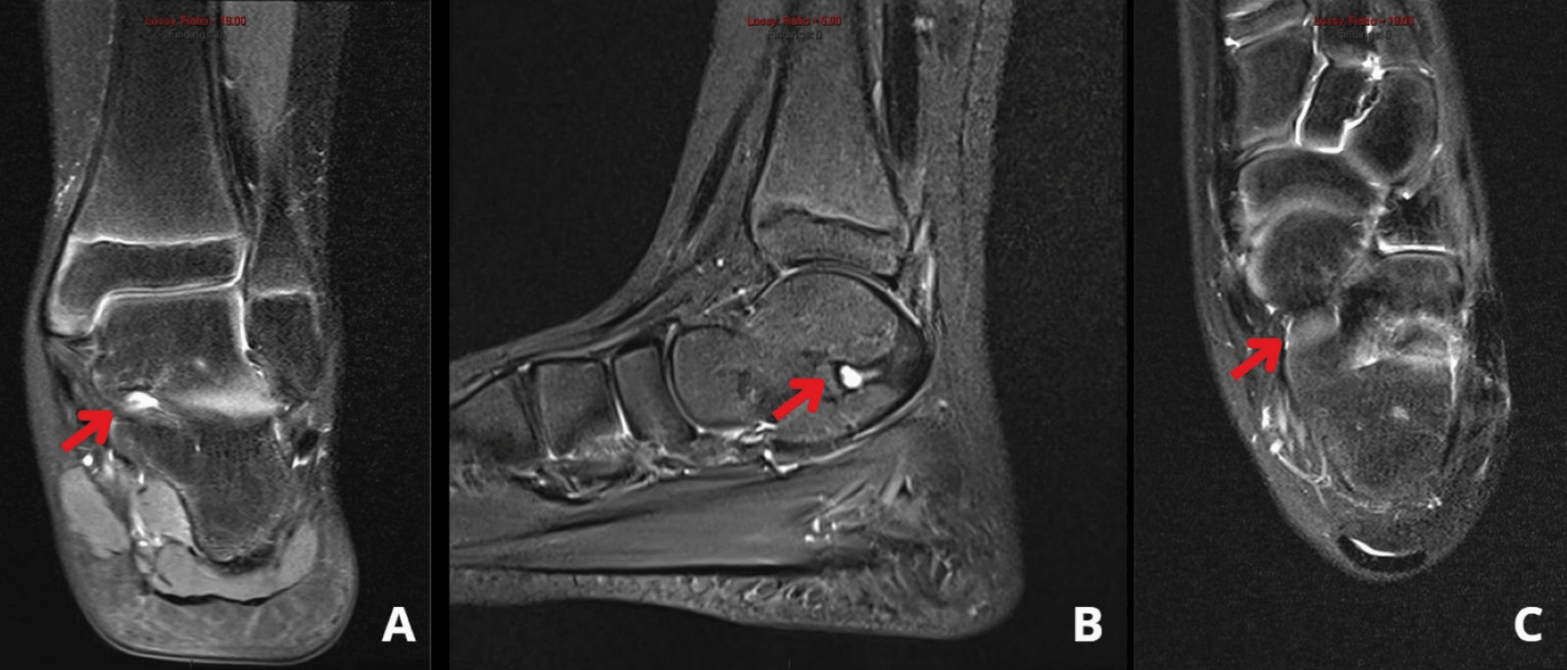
Magnetic resonance imaging of the left foot demonstrating (A) a coronal view, (B) a sagittal view, and (C) a transverse view, revealing a fibrous talocalcaneal coalition (red arrow).
